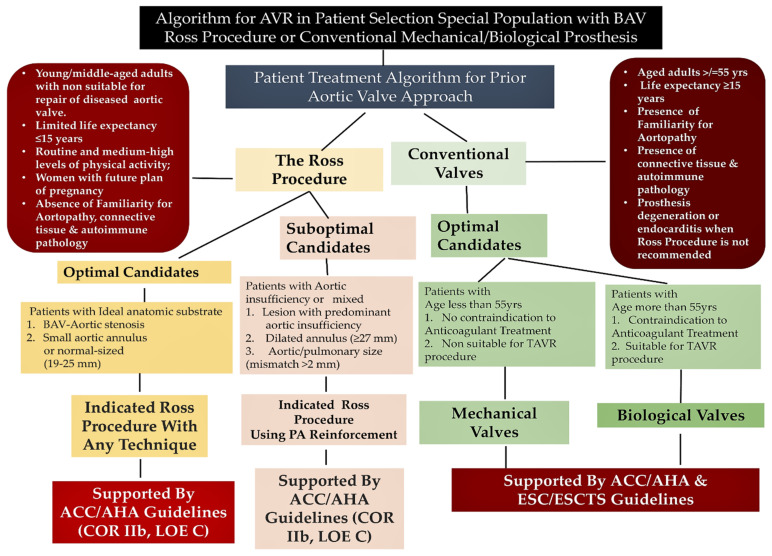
Algorithm for patient special population selection for aortic valve replacement. Ross procedure or conventional mechanical/biological prosthesis may be used according with international guidelines. Abbreviations; ACC, American College of Cardiology; AHA, American Heart Association; BAV, bicuspid aortic valve; COR, class of recommendation; ESCTS, European Society of Cardiothoracic Surgery; ESC, European Society of Cardiology; LOE, level of evidence; TAVR, transthoracic aortic valve replacement.
